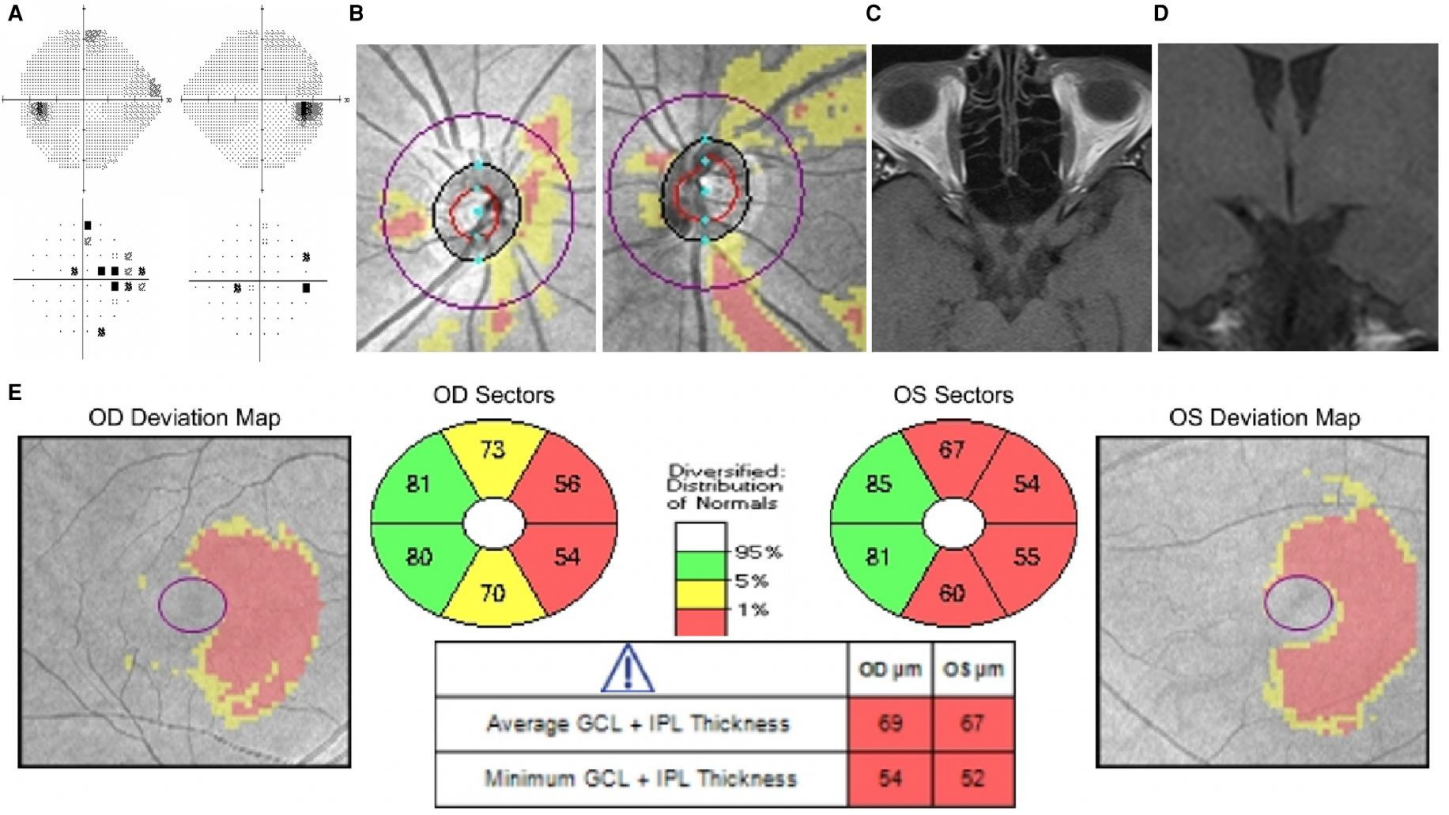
Left OTS. (A) Very subtle right HH seen on greyscale map and pattern deviation map, (B) band atrophy of the right optic disc and hourglass atrophy of the left optic disc, (C) thinning of the left optic tract seen on MRI axial T1 image and (D) coronal T1 image. (E) Left homonymous thinning of the GCL.
